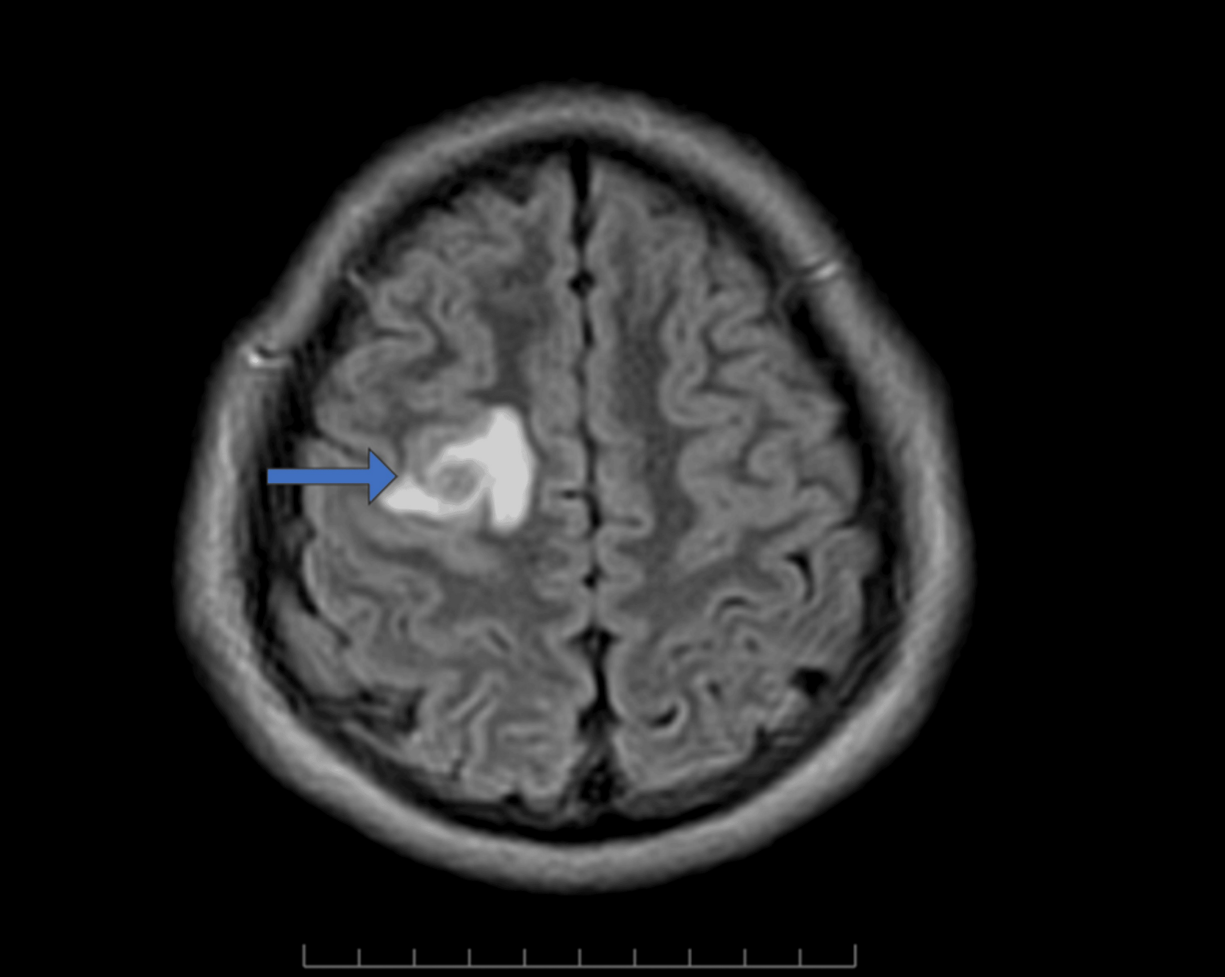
Axial FLAIR MRI image showing a central hypointense lesion with surrounding perilesional hyperintense edema (arrow). FLAIR, fluid-attenuated inversion recovery
